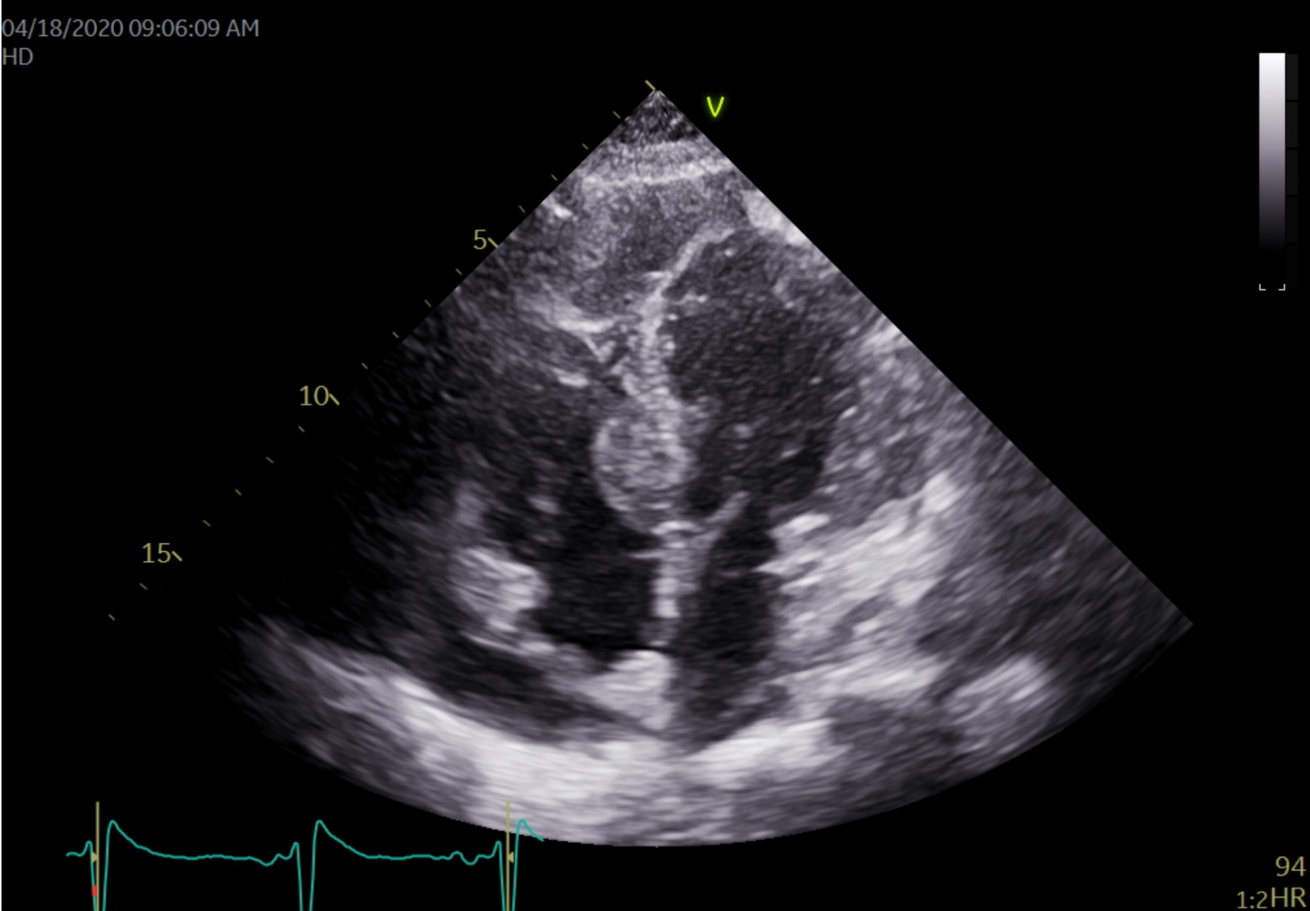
An apical 4 chambers view revealing the pericardial effusion present with a 1.62 cm x 2.45 cm mass in the basal septal portion of the right ventricle
